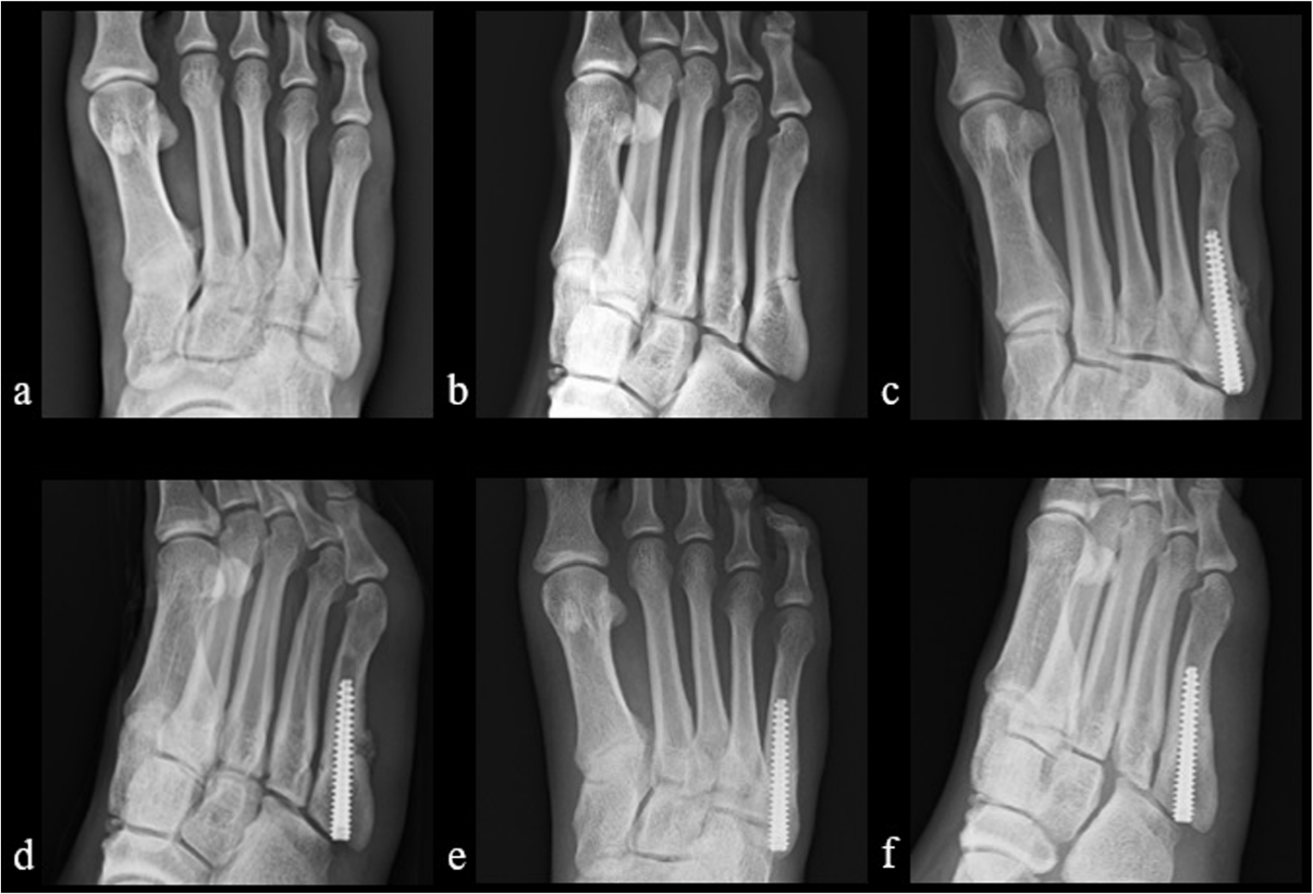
Case presentation. A 19-year-old patient without LIPUS treatment after intramedullary screw fixation. a and b. Radiographs showed proximal metatarsal stress fracture preoperatively (a. anterior-posterior and b. oblique view). c and d. Radiographs in the immediate postoperative period (c. anterior-posterior and d. oblique view). e and f. The radiographs obtained 12 weeks postoperatively showed bone union (e. anterior-posterior and f. oblique view)
